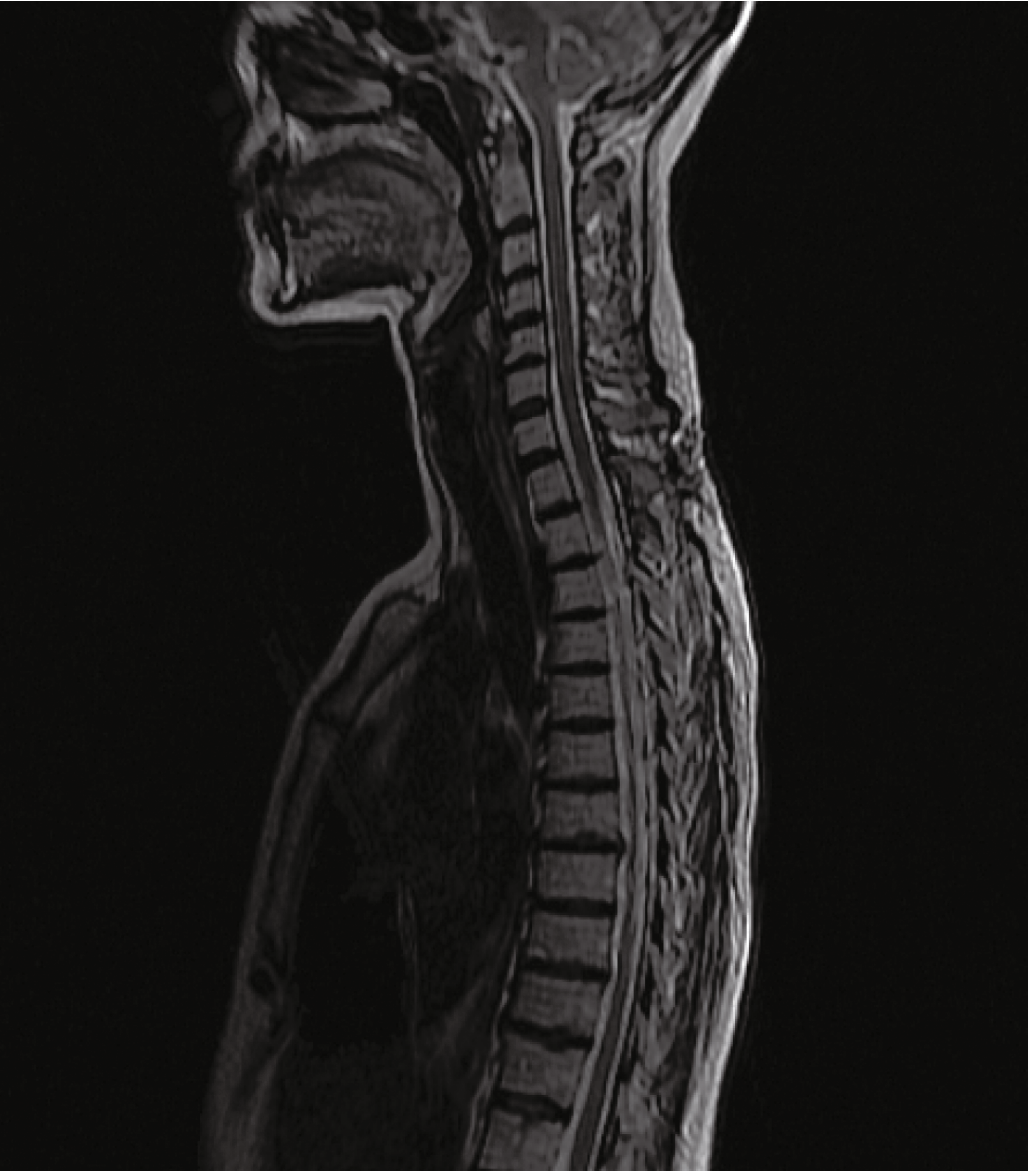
Sagittal T_2_-weighted magnetic resonance imaging of the thoracic spine after surgery.
